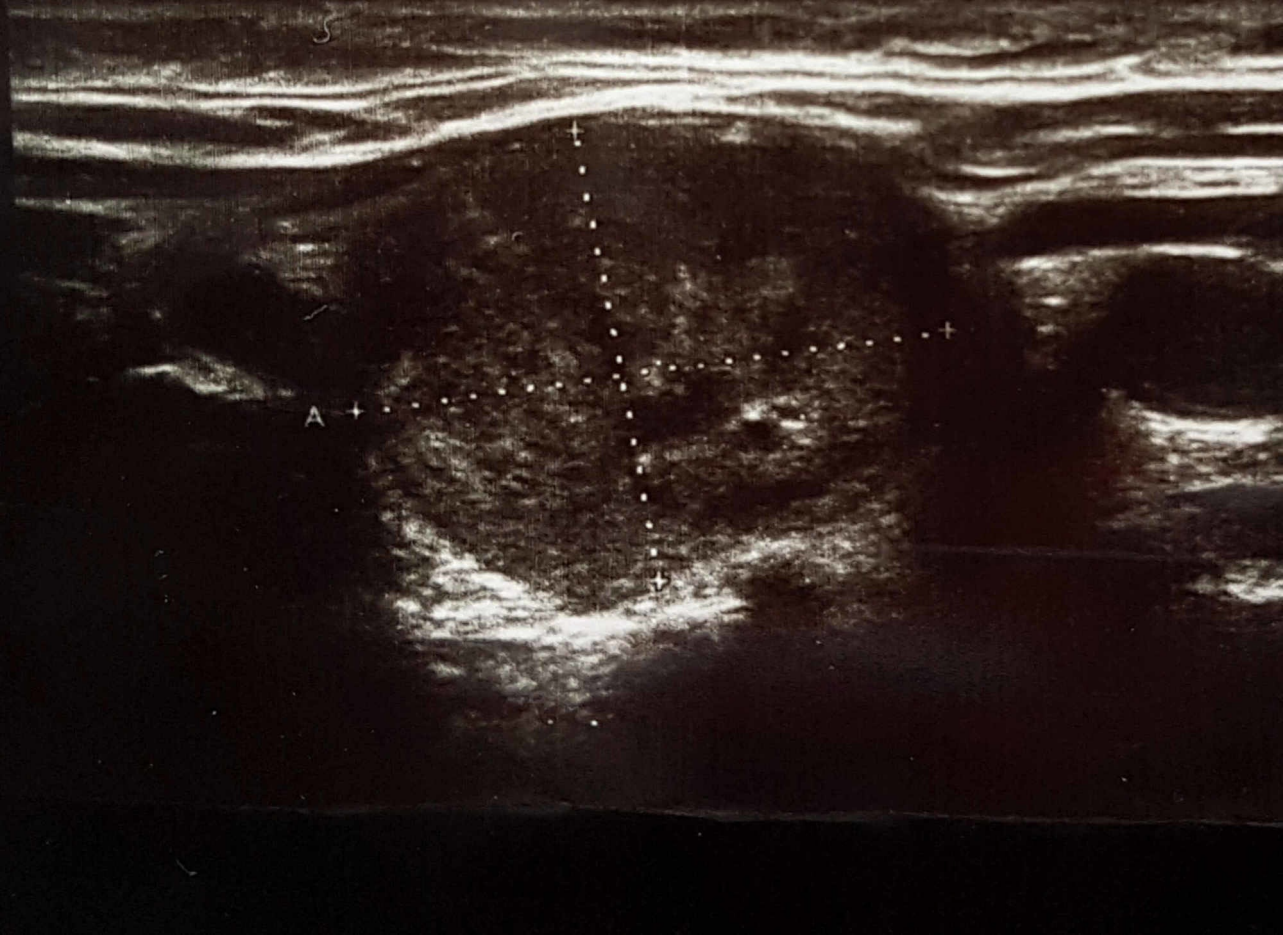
Right lobe ultrasound Ultrasound shows a heterogeneous solid nodule that is a functioning "hot" nodule in thyroid scintigraphy.
